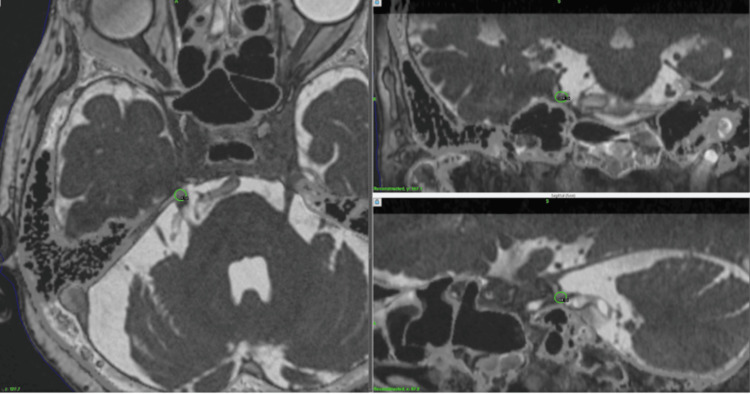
Dose planning for Case 1 MRI (left, axial image; upper right, coronal; lower right; sagittal) on GammaPlan (Elekta, Tokyo) workstation. Radiosurgery shot placement was indicated in green circles, in each image. MRI, magnetic resonance images
